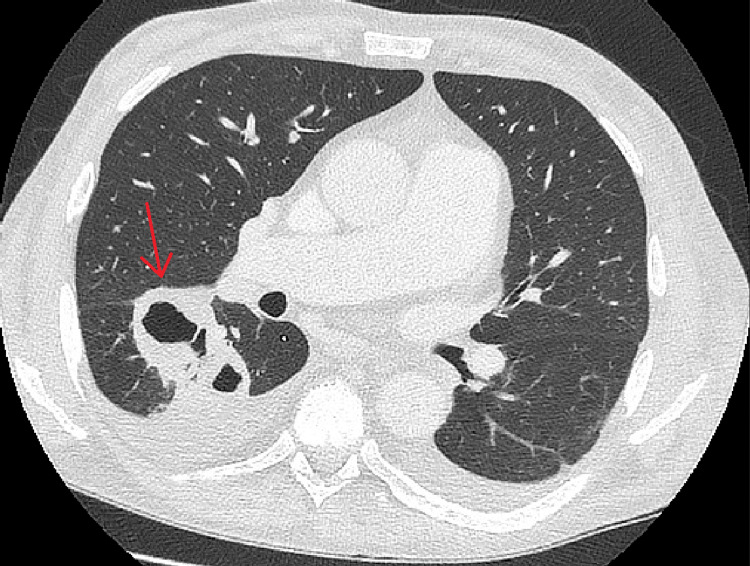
Chest computed tomography with visible lesions in segment VI of the right lung (red arrow) at the initiation of the treatment (July 2024).
